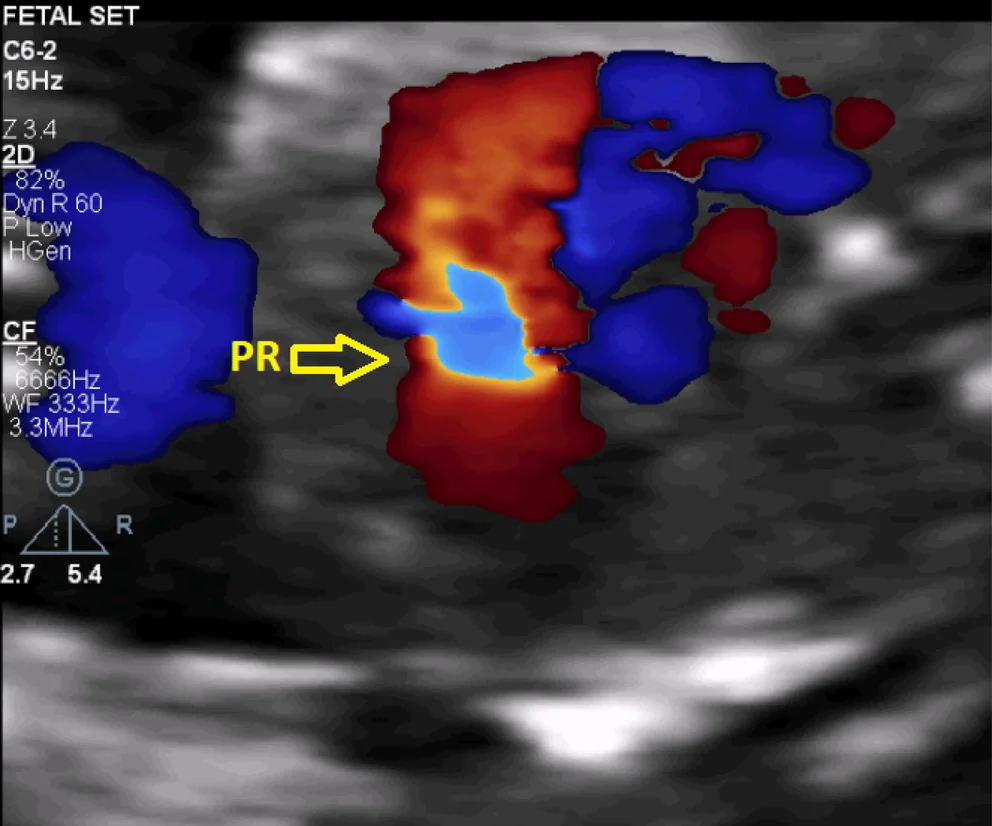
Color Doppler imaging in the axial view demonstrates significant pulmonary regurgitation (PR) with diastolic retrograde flow in the main PA.
